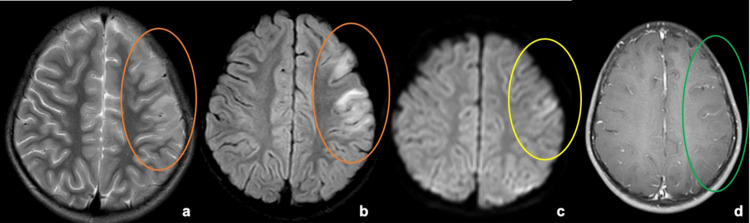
MRI brain sections at the level of the centrum semiovale From left to right: (a) T2W, (b) FLAIR, (c) DWI (d) Post-contrast T1W images. Areas within the orange ellipse in (a) and (b) show focal T2/FLAIR hyperintensities in the left frontal cortical and juxta/subcortical regions with gyral swelling and sulcal effacement. The yellow ellipse marked in the DWI image (c) shows corresponding areas of cortical restriction. There is a thin leptomeningeal enhancement in the areas corresponding to sulcal effacement as marked with the green ellipse in (d). DWI - Diffusion-weighted imaging; FLAIR - Fluid attenuated inversion recovery; MRI - Magnetic resonance imaging; T1W - T1 weighted; T2W - T2 weighted
